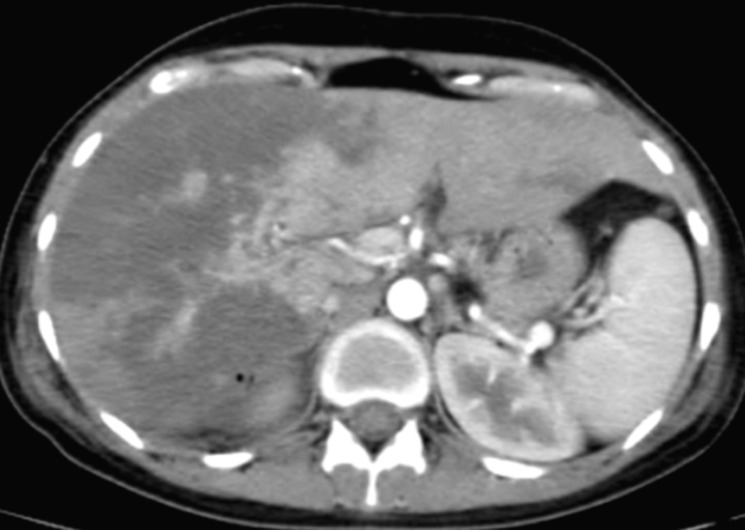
contrast-enhanced CT of the abdomen showing the presence of diffuse hypodense plaques more marked on the right hepatic lobe consistent with massive hepatic infraction
